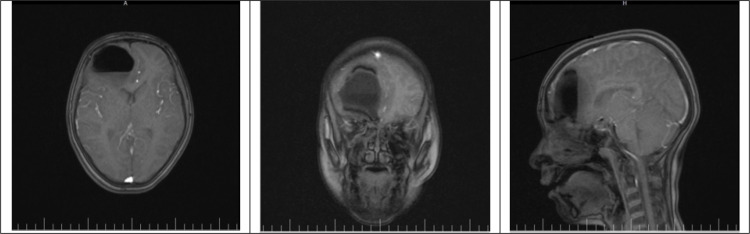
Magnetic resonance imaging (MRI) with contrast (Axial, coronal and sagittal views) revealed no rim enhancement around the lesion, which ruled out brain abscess and confirmed tension hydropneumocephalus diagnosis
